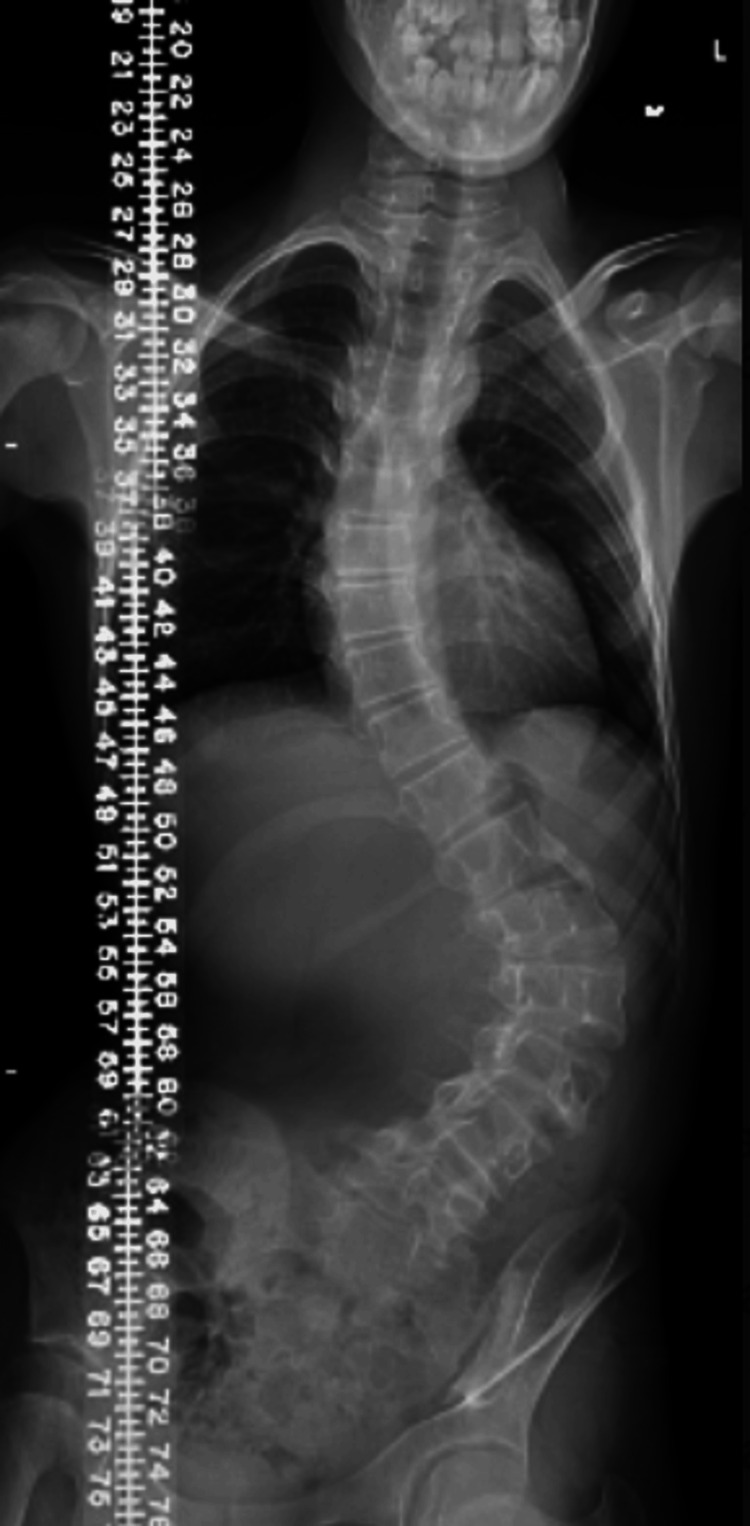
Radiograph of the thoracolumbar spine with S-shaped scoliosis.
